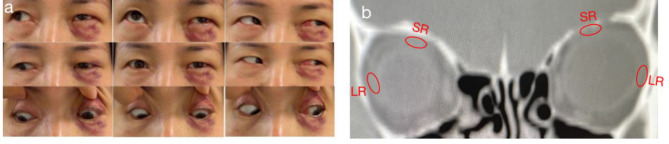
Clinical photographs and CT scan of the patient showing ocular alignment after surgery. a Orthotropia on the postoperative day 7 b Orbital CT showed dislocated globe back into the muscle cone
